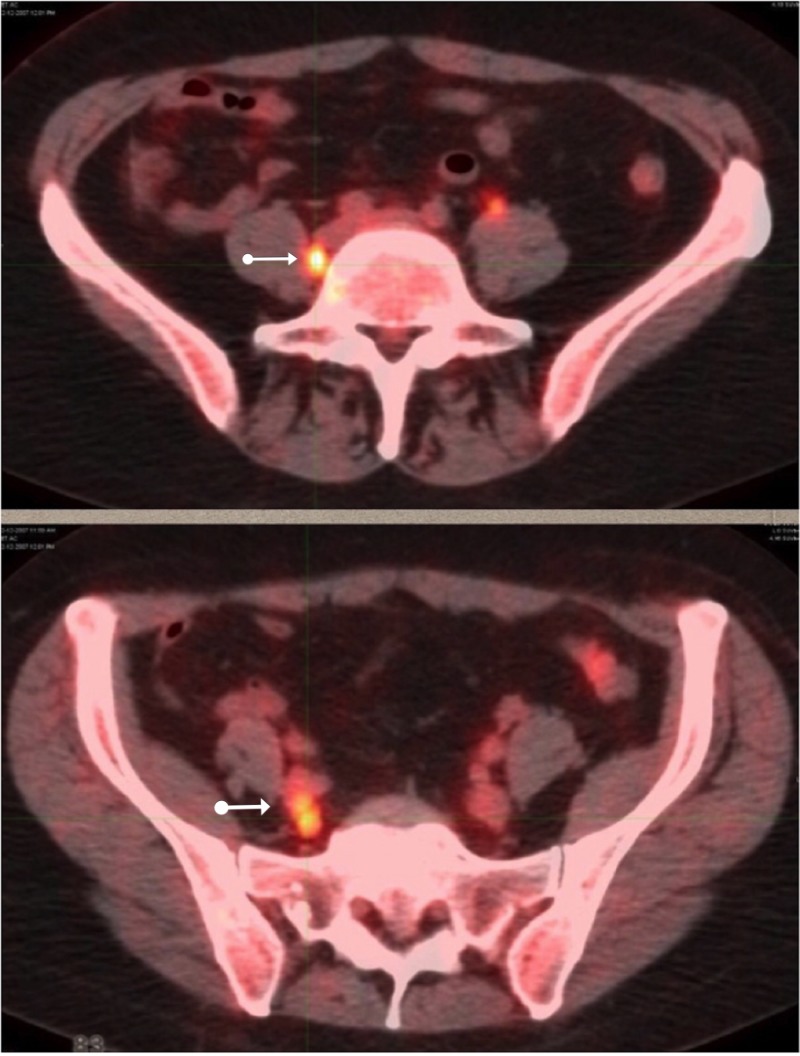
PET/CT three months post-treatment positron emission tomography-computed tomography: PET/CT
